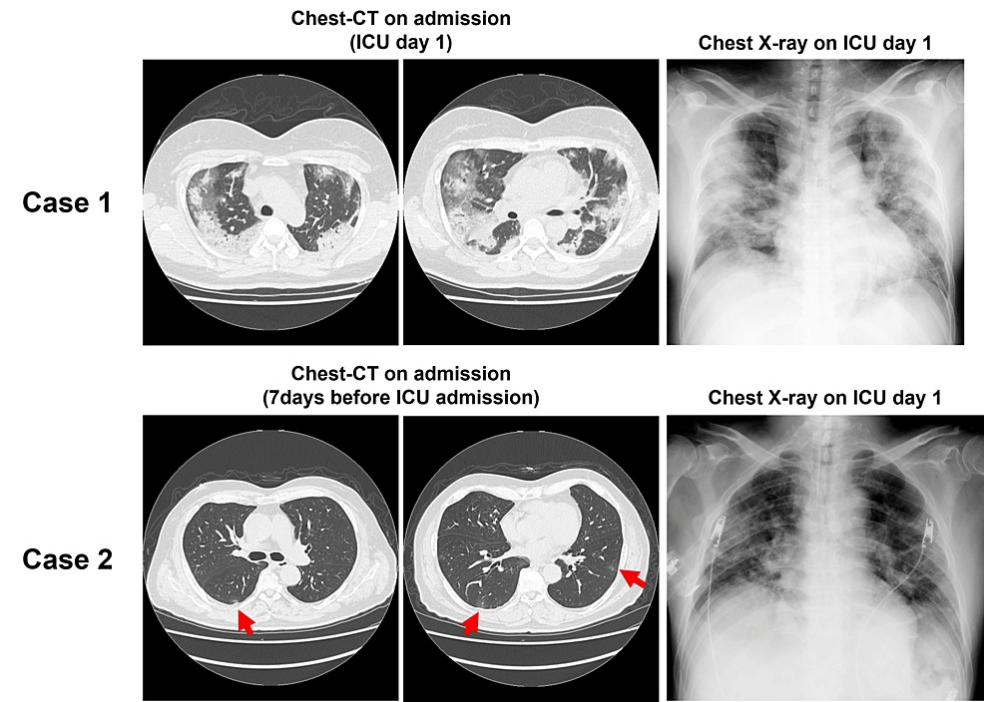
The images of chest computed tomography (CT) on admission and chest X-ray on ICU day 1 In the chest-CT images of case 2, red arrows indicate bilateral and multiple ground-glass opacities.
